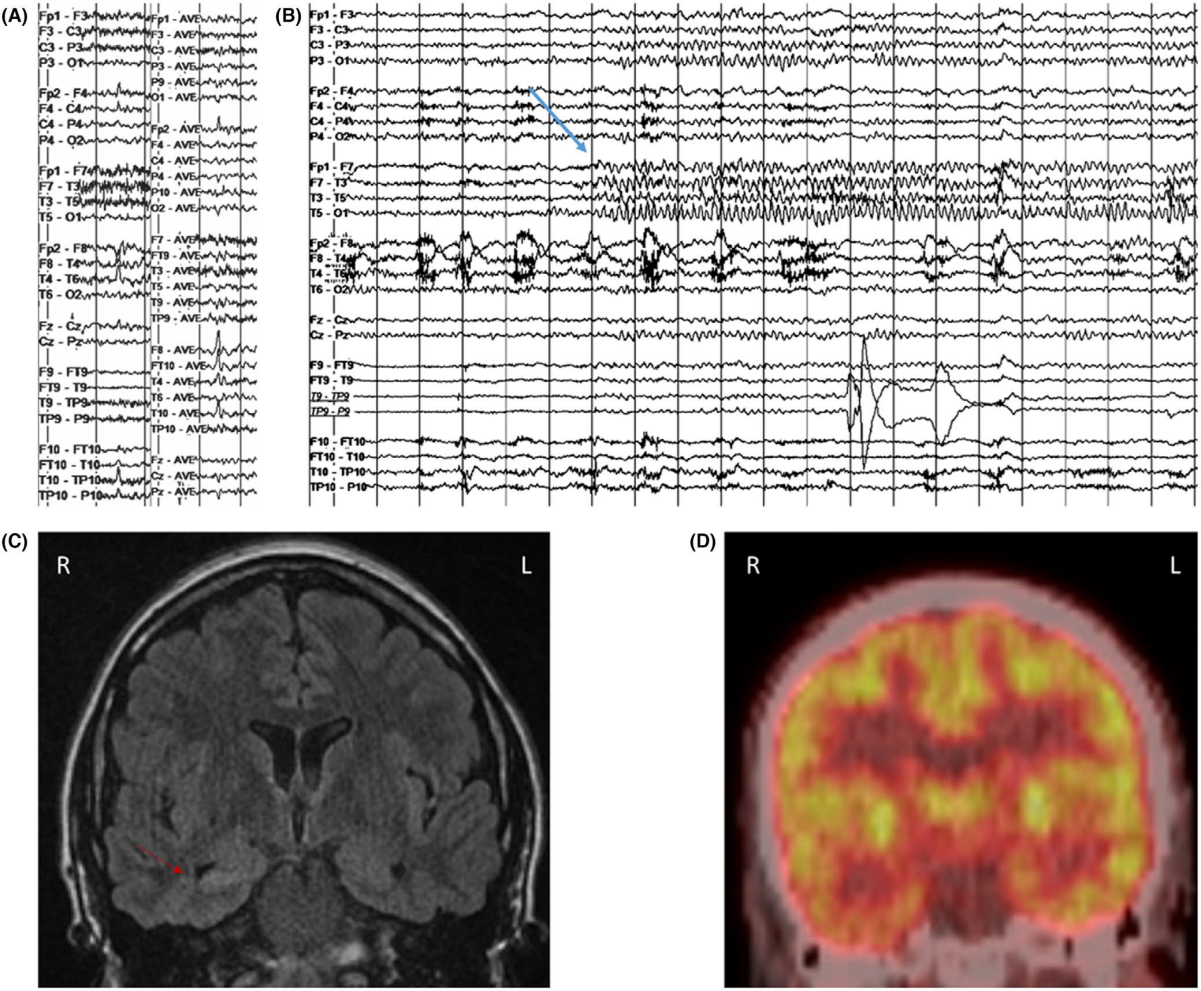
Phase I work‐up in a 27‐year‐old right‐handed male with a family history of seizures in his maternal grandmother. Epilepsy onset was at age 19 years with focal impaired consciousness seizures occurring multiple times per month despite multiple antiseizure medications. Seizures were recorded as episodes of behavioral arrest with orolingual automatisms characterized by lip smacking followed by postictal fatigue. He did not have postictal aphasia. (A) Interictal EEG showed frequent right anterior to mid‐temporal spikes (maximum negativity over F8 > FT10 > T10) (1–70 Hz bandpass, bipolar longitudinal montage with subtemporal chains). Clinical onset (aura) preceded electrographic onset, with (B) first ictal changes on scalp EEG characterized by rhythmic sinusoidal 5–6 Hz theta activity over the left hemisphere maximal over the left temporal region (arrow) (20s, 1–70 Hz bandpass, bipolar longitudinal montage with subtemporal chains). (C) 3 T Brain MRI showed reduced right hippocampal volume with hyperintense T2 signal consistent with right mesial temporal sclerosis, and (D) FDG‐PET showed right temporal hypometabolism. Neuropsychometric testing was largely normal with preserved left mesial temporal function. In summary, there was a discrepancy between scalp EEG seizure onset in the left temporal region and a lesion, irritative zone (interictal discharges), and functional deficit zone (FDG‐PET) in the right mesial temporal lobe. Additionally, seizure semiology suggested a symptomatogenic zone within the mesial temporal lobe in the absence of definitive lateralizing features, and the patient did not have a focal neuropsychological deficit. As a result, a phase II evaluation with stereoelectroencephalography (SEEG) monitoring was recommended to test the hypothesis for the epileptogenic zone (Figure 13). His 5‐SENSE score suggested that SEEG is likely to identify a focal seizure onset zone. Computation of his Specific Consistency Score suggests a score of 6 (grade B) which indicates that his data were partially consistent and further supports the decision for invasive monitoring.
